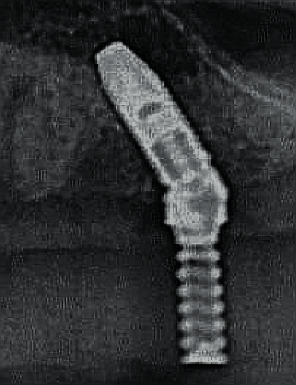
Radiographic control of the angled multiunit base fit into the implant.
